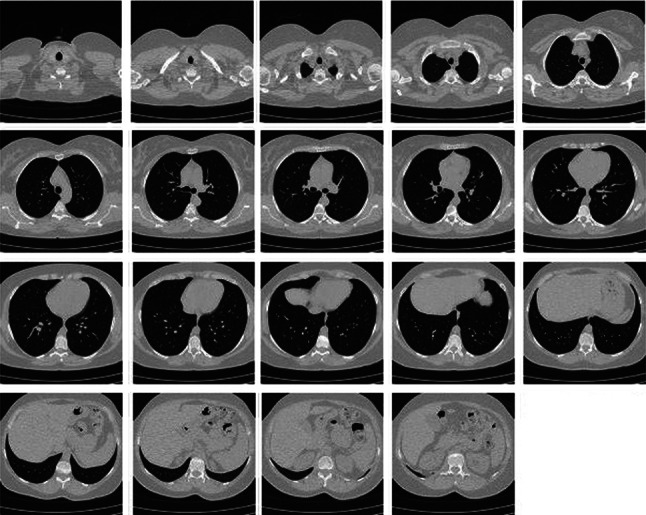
Sample images from each class of EXACT09-CT database
